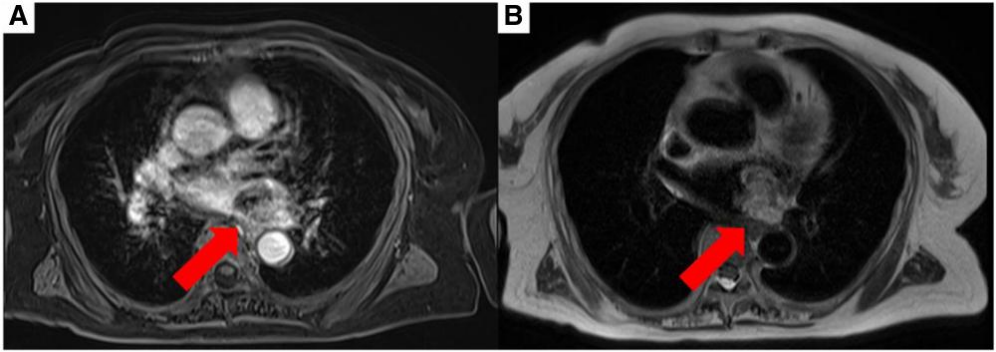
Contrast-enhanced cardiac magnetic resonance imaging findings. (*A*) T1-weighted imaging shows predominantly low signal intensity with areas of higher intensity. (*B*) T2-weighted imaging shows high signal intensity interspersed with low-intensity regions, suggesting internal heterogeneity.
